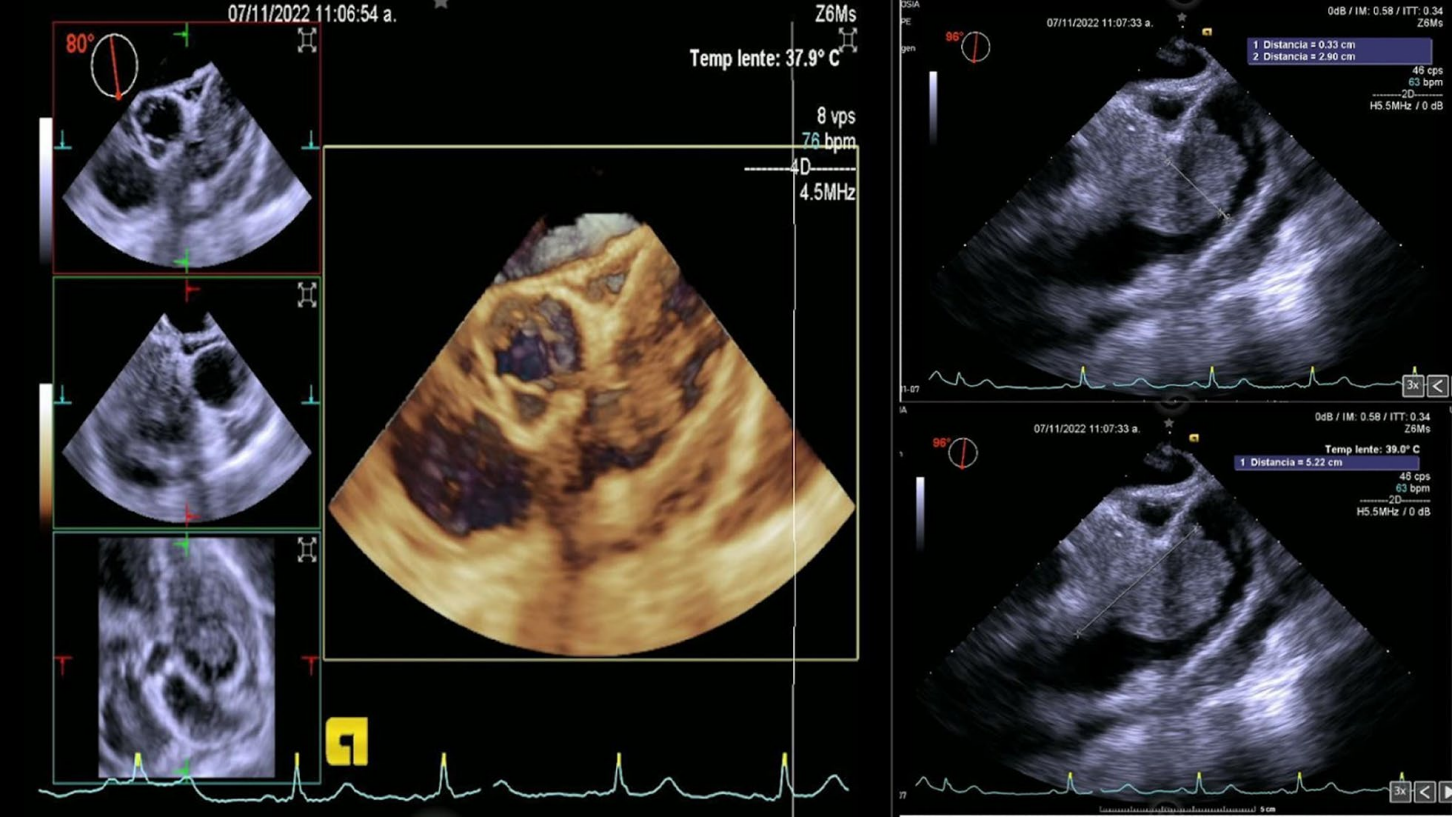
Transesophageal echocardiogram. A tumor mass fixed to the anterior wall of the trunk of the pulmonary artery
